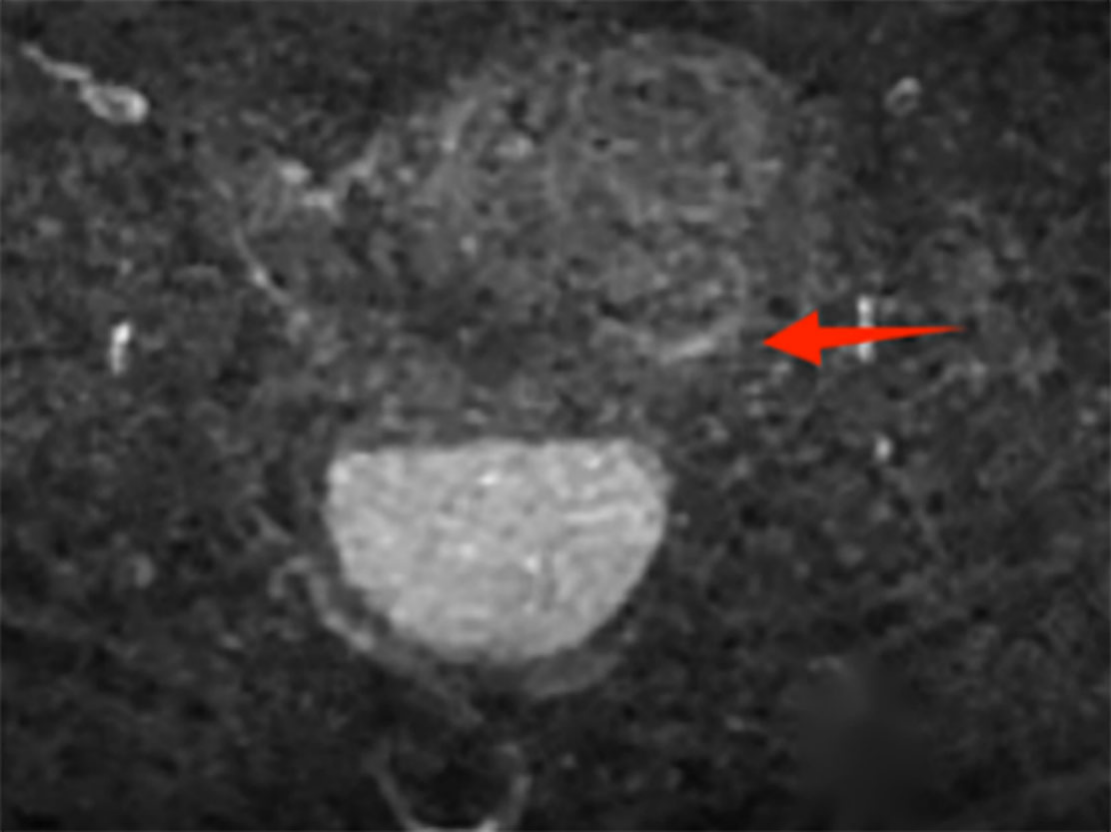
Post-drainage follow-up MRI T2-weighted imaging demonstrates stratification of cystic fluid; the left lateral cervical wall shows focal mild diffusion restriction, with hyperintense signal on DWI.
